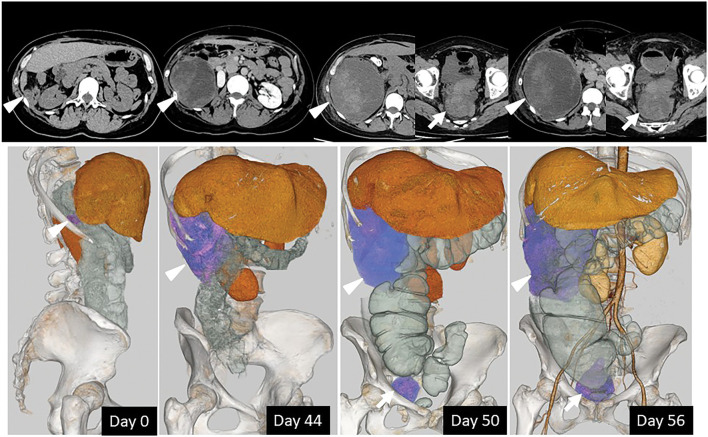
Abdominal CT and 3D reconstructed images of disseminated lesions. The arrowheads indicate the peritoneal lesion along the right paracolic gutter, and the arrows indicate the lesion in the pouch of Douglas; the same symbols correspond to identical lesions on both CT and 3D reconstructed images. Bowel obstruction due to an enlarged lesion was observed. The number of days since the date when peritoneal dissemination was first identified is annotated within the 3D images.
